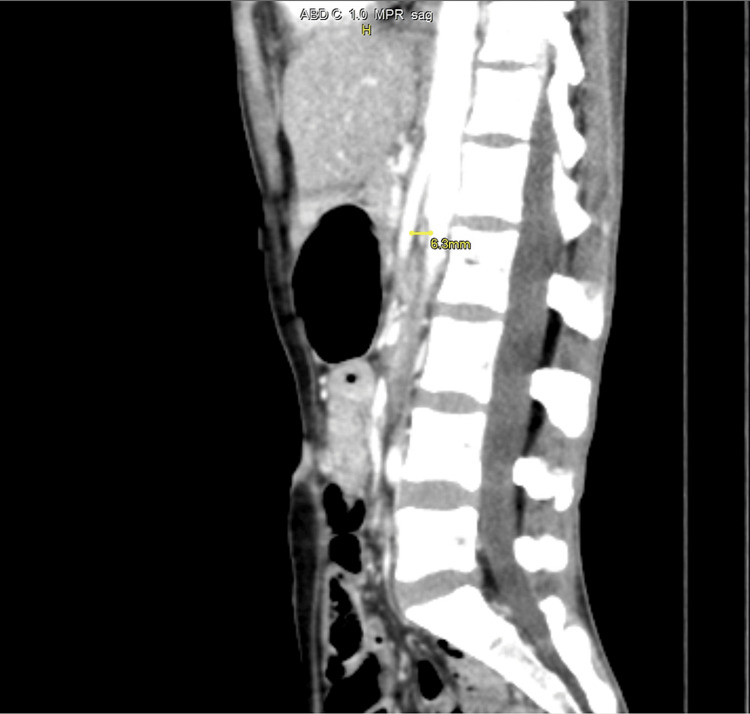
The image depicts an AOM distance measuring 6.3 mm. This is the sagittal section of the contrast-enhanced MRI of the abdomen. AOM, aortomesenteric
